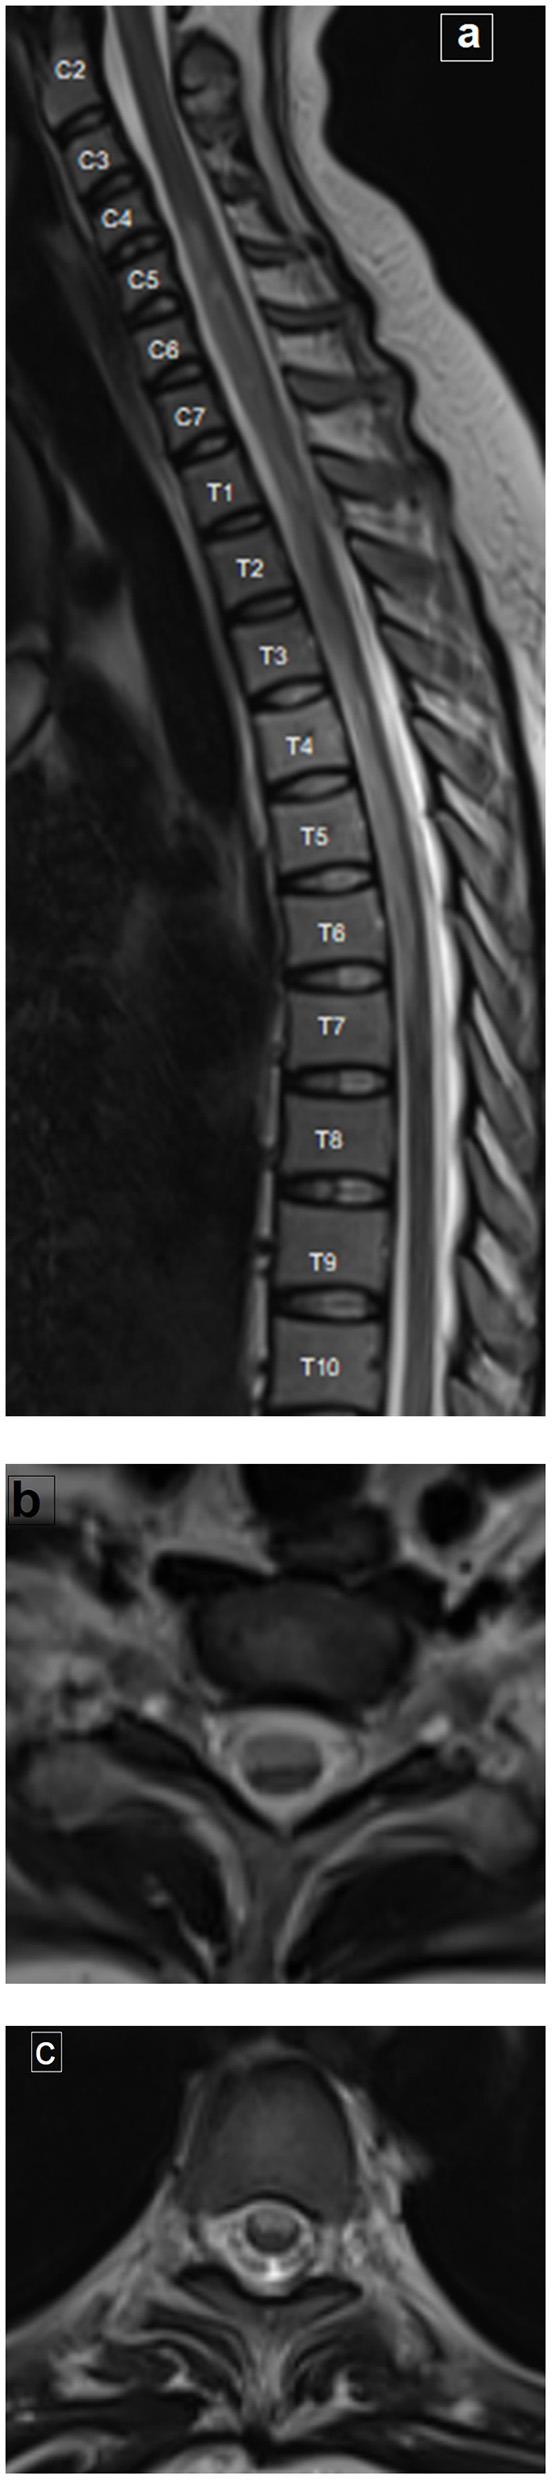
MRI of the spine. (a) Sagittal T2-weighted image of the whole spine shows extensive long hyperintense signal extending from C5 to T7, associated with mild cord swelling. (b, c) Axial T2-weighted images at the cervical and dorsal levels, respectively, showing the hyperintense signal occupying the anterior two thirds of the cord, bilaterally (with involvement of both the gray and white matter).
